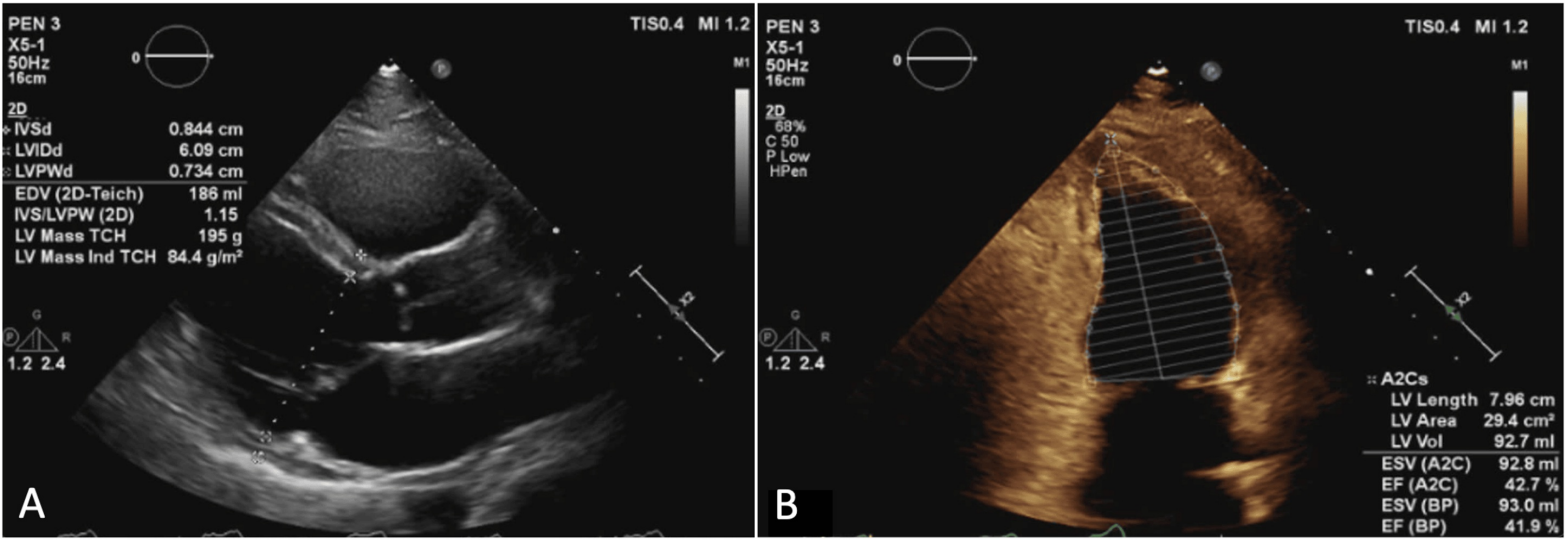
Parasternal long-axis (A) and two-chamber view (B) TTE demonstrate a dilated left ventricular cavity with an end-diastolic diameter measuring 6.1 cm; however, relative wall thickness and mass index are normal. The LVEF is 42% by Simpson’s biplane method. TTE: transthoracic echocardiography; LVEF: left ventricular ejection fraction
